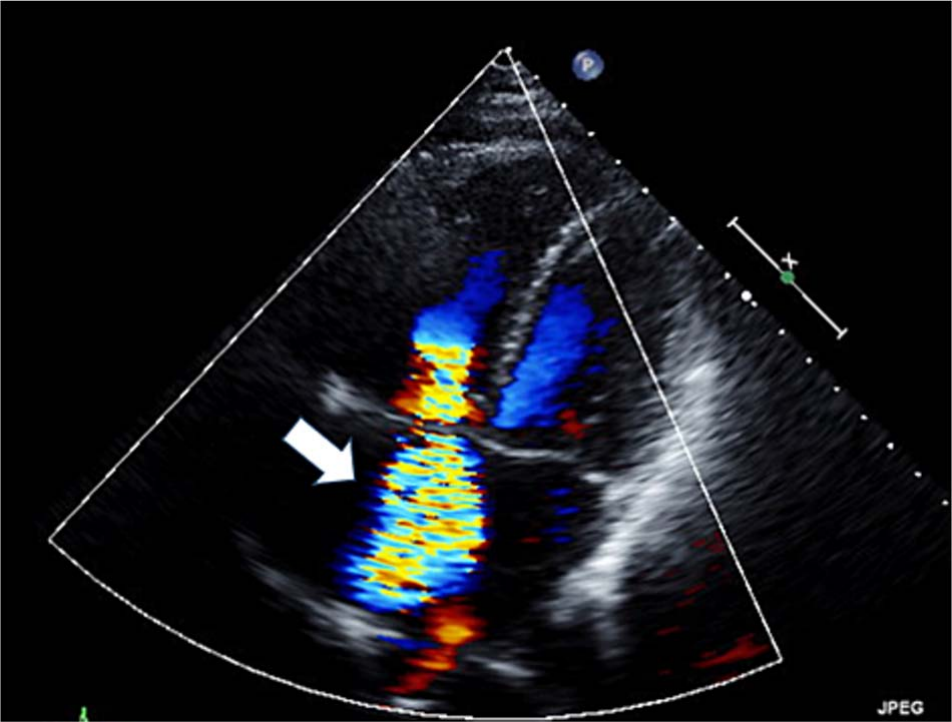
Preoperative transthoracic echocardiography: severe tricuspid regurgitation. The regurgitant jet is indicated by the white arrow.
